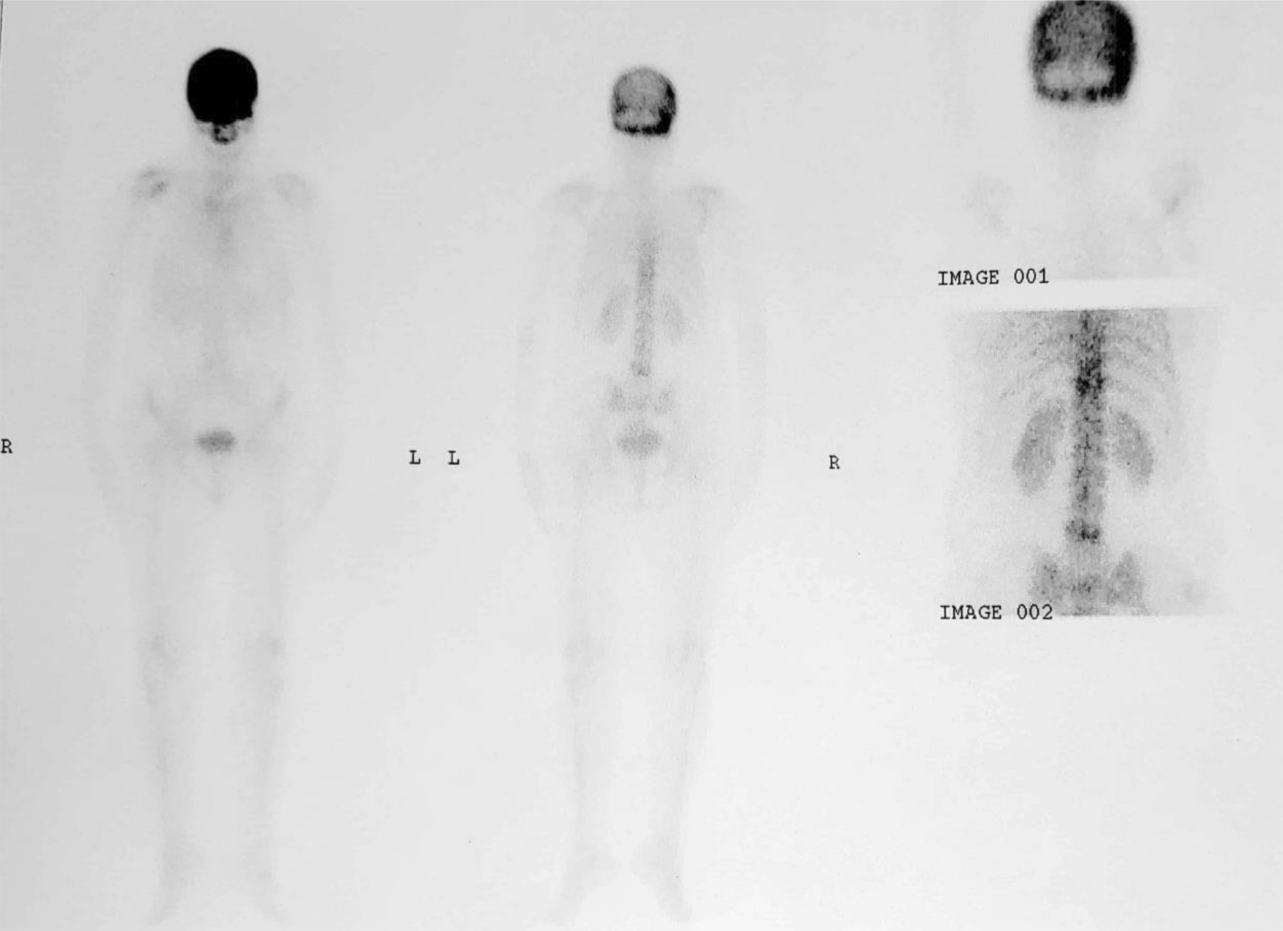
Bone scintigraphy by Tc‐99m‐MDP showing increased tracer activity in the skull, T11 and L5 vertebrae which is highly suggestive of Paget's disease of bone. Although Tc‐99m‐MDP is highly sensitive but not specific. It is useful to define the overall extent and distribution of disease.
